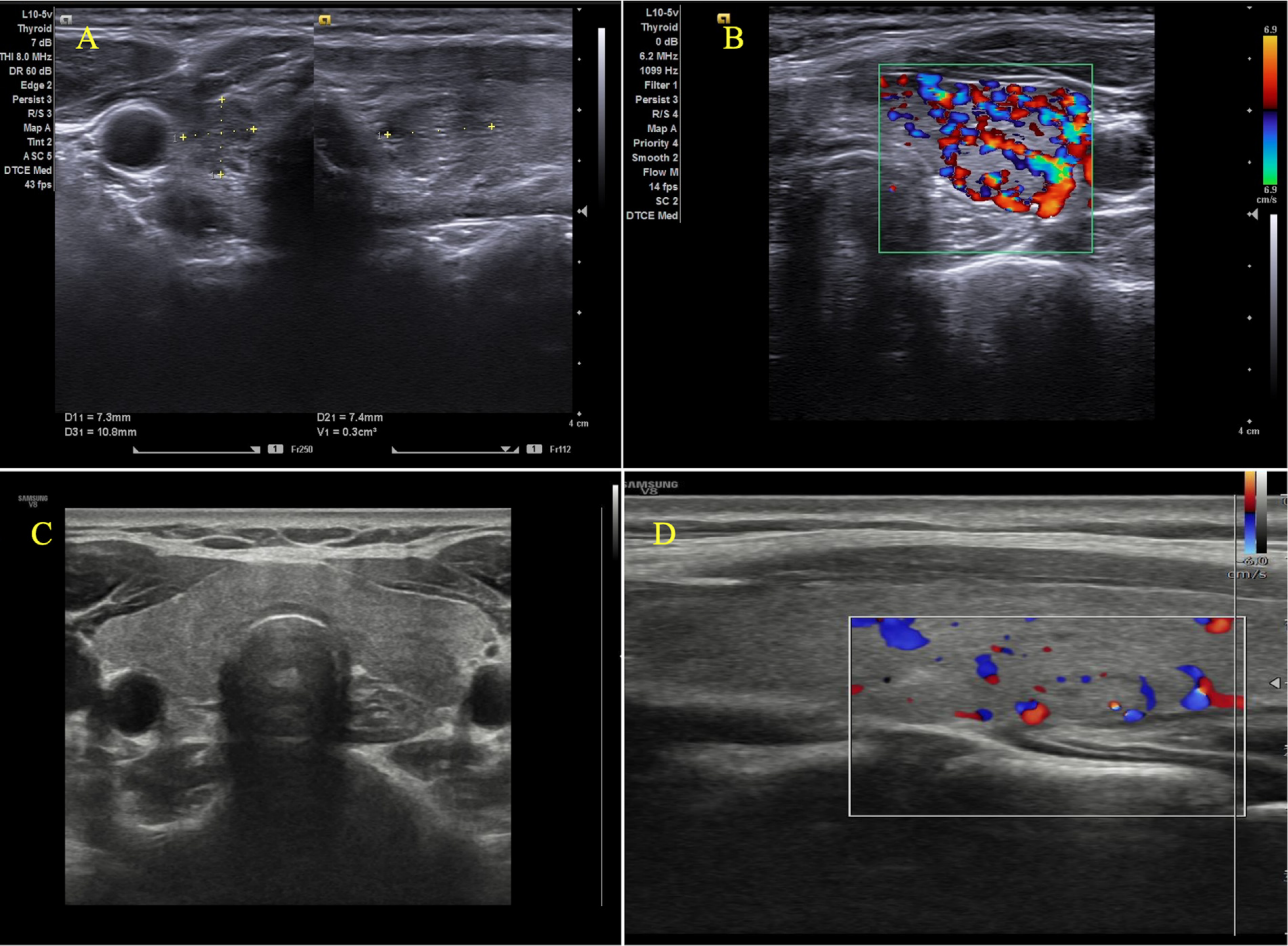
(A, B) Six months post-ablation, ultrasound demonstrates heterogeneous echogenicity in the thyroid gland with markedly increased vascularity on Color-Doppler imaging. (C, D) At 12 months post-ablation, ultrasound reveals homogeneous echogenicity of the thyroid gland with no signs of tumor recurrence.
